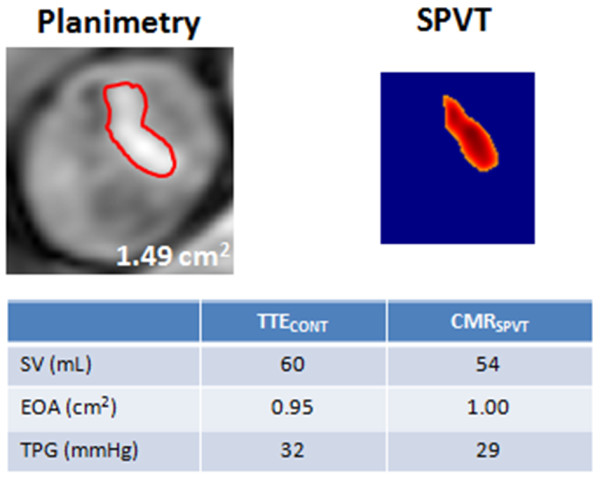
Example of a patient with discordant echocardiography findings in whom stenosis severity was corroborated by the measurement of EOA with the use of CMR_SPVT_. EOA: effective orifice area; SV: stroke volume; TPG: transprosthetic gradient.
